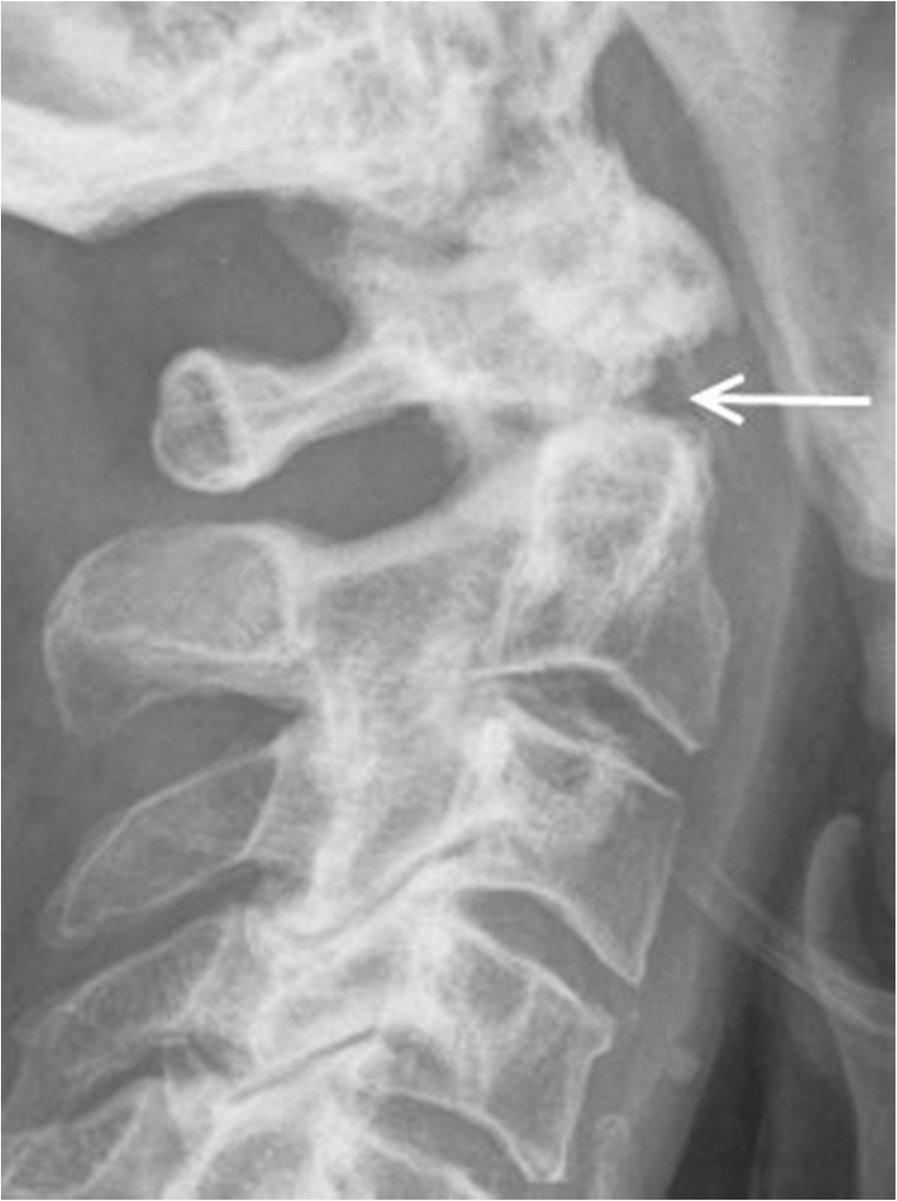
Lateral radiographs obtained at 3-year follow up in a 77-year-old man who suffered a type II odontoid fracture after a car accident and was treated non-operative. This image demonstrate lack of fusion.
